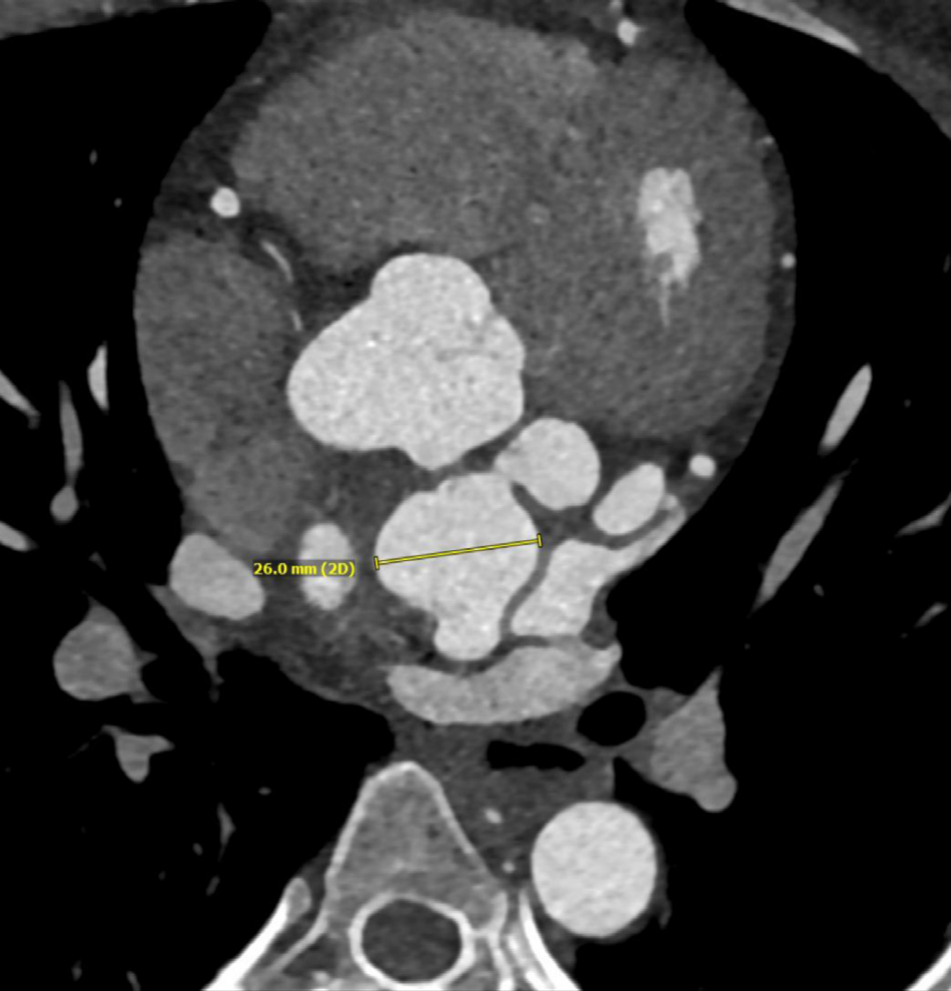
Contrast-enhanced axial CTA image: aneurysmal dilation of the LCX CAF measures up to 26 mm (ruler measurement).
